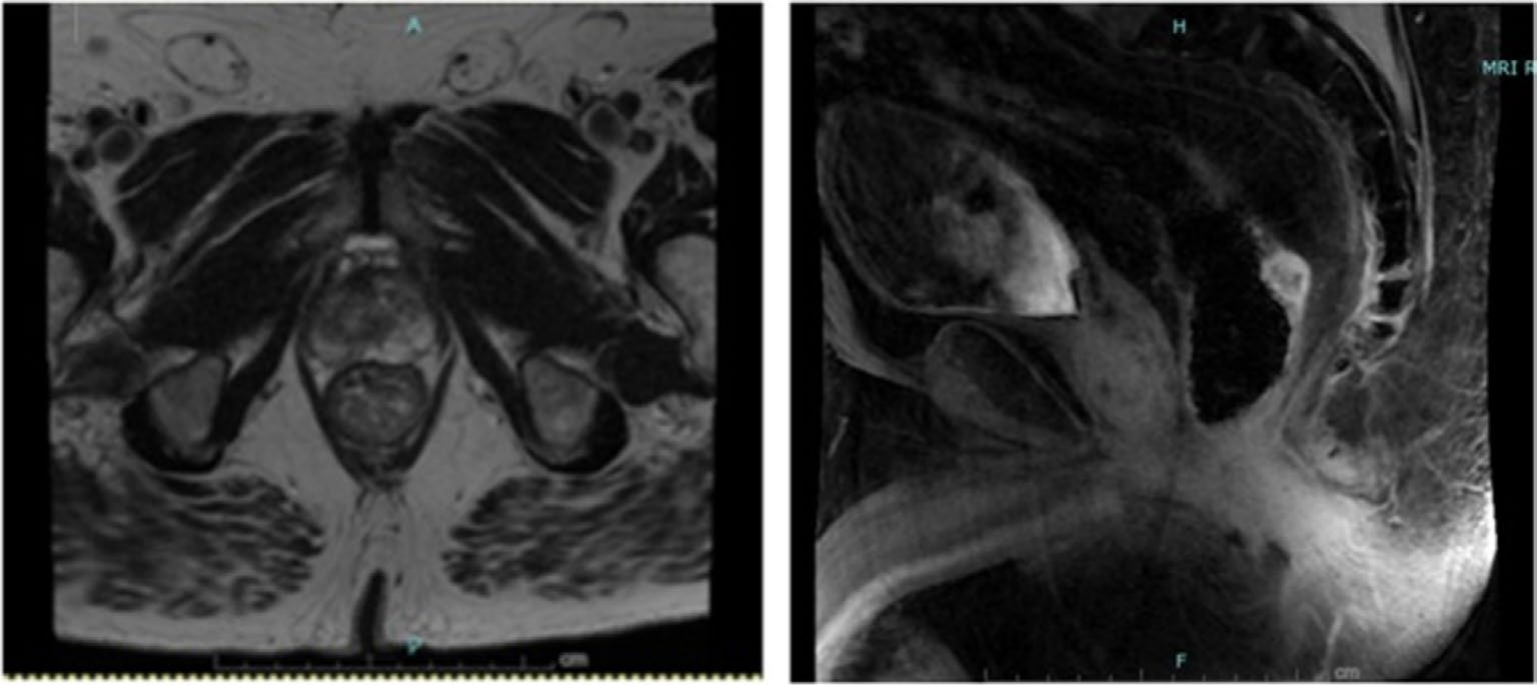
MRI image of tumor recurrence.
